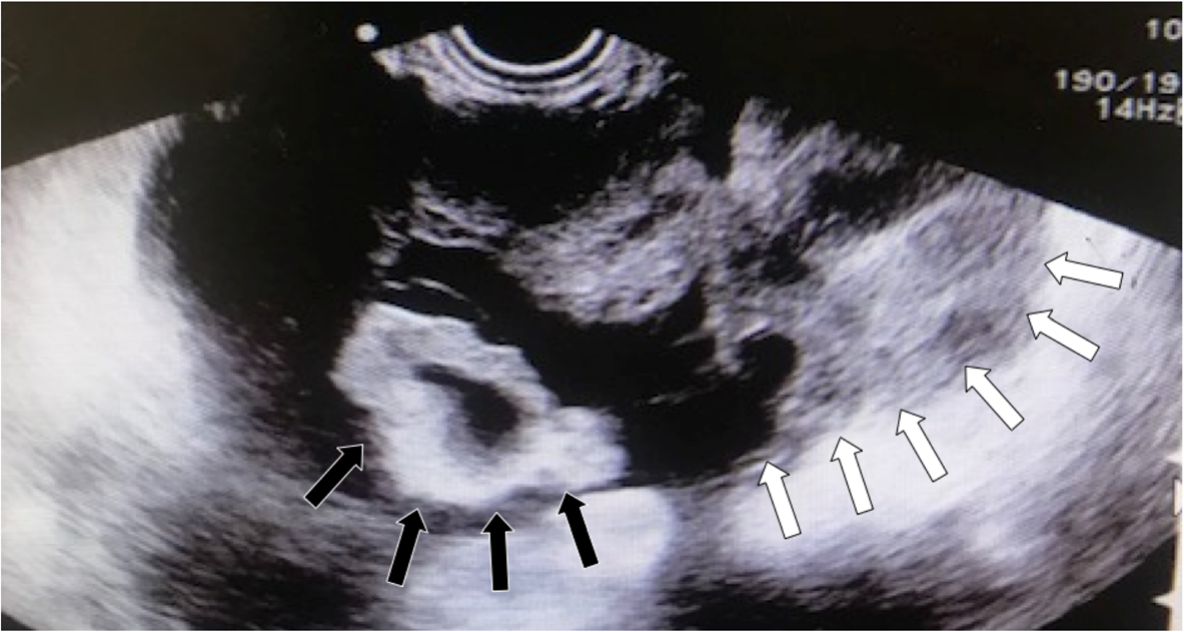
Findings by transvaginal ultrasonography. The *white arrows* indicate the enlarged right ovary; the *black arrows* indicate the fallopian tube, which was believed to have enlarged due to the adjacent hollow structure
